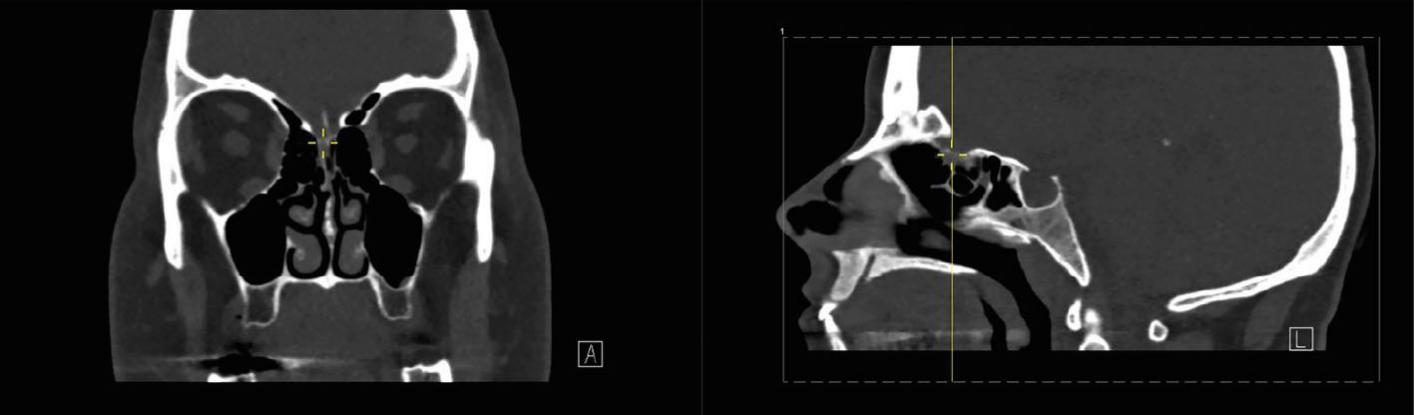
Preoperative coronal and sagittal CT images of a possible CSF leakage site at the right lateral lamella of the cribriform plate with no obvious (meningo)encephalocele.
